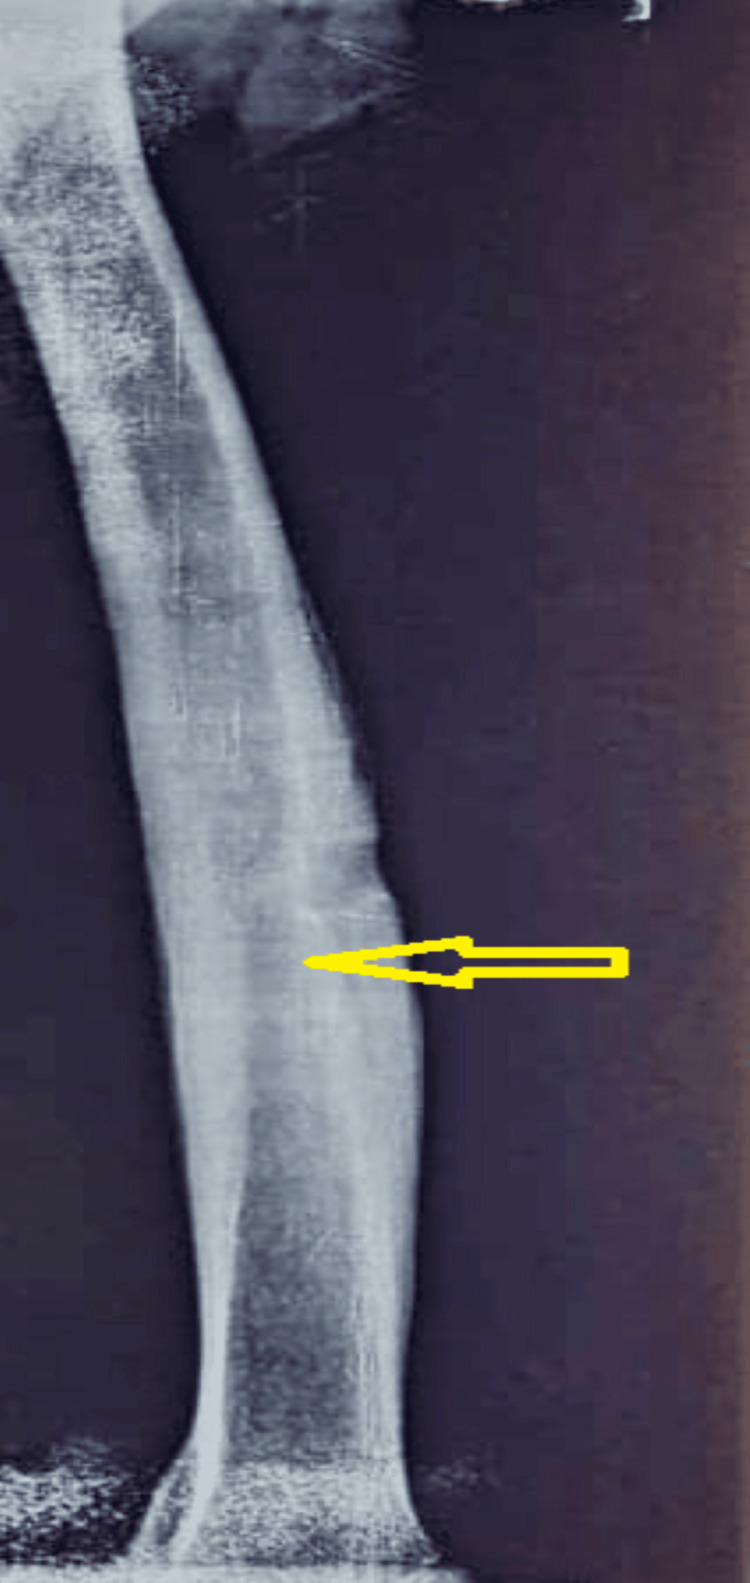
Follow-up radiograph of the left femur obtained one year post-treatment The anteroposterior view demonstrates significant cortical remodeling and progressive ossification of the previously noted lytic regions. There is a marked reduction in periosteal thickening and normalization of the medullary density compared to the initial presentation. No new lytic lesions, sequestra, or radiographic signs of fungal recurrence are identified (yellow arrow), confirming a successful long-term response to combined surgical debridement and antifungal therapy.
